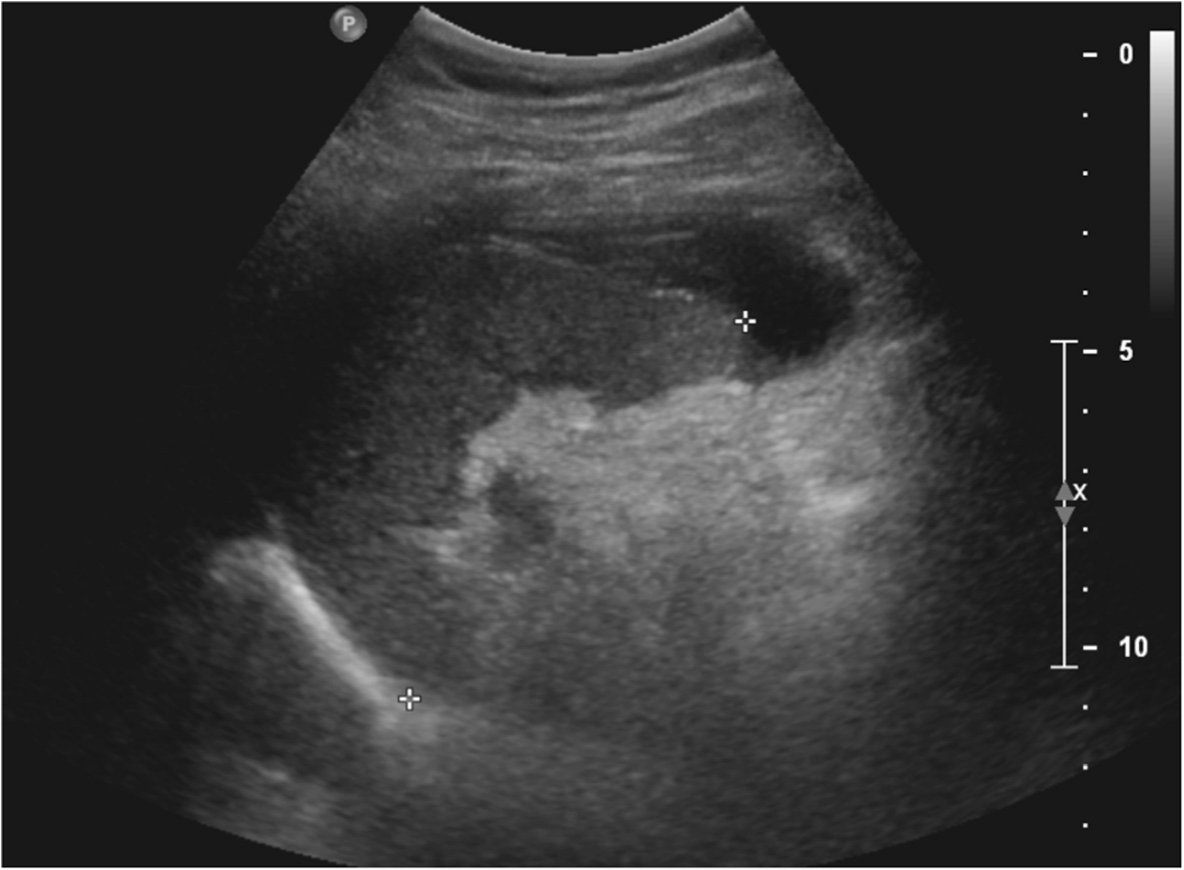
2D gray scale ultrasound image demonstrates small degree of subcapsular splenic hematoma.
